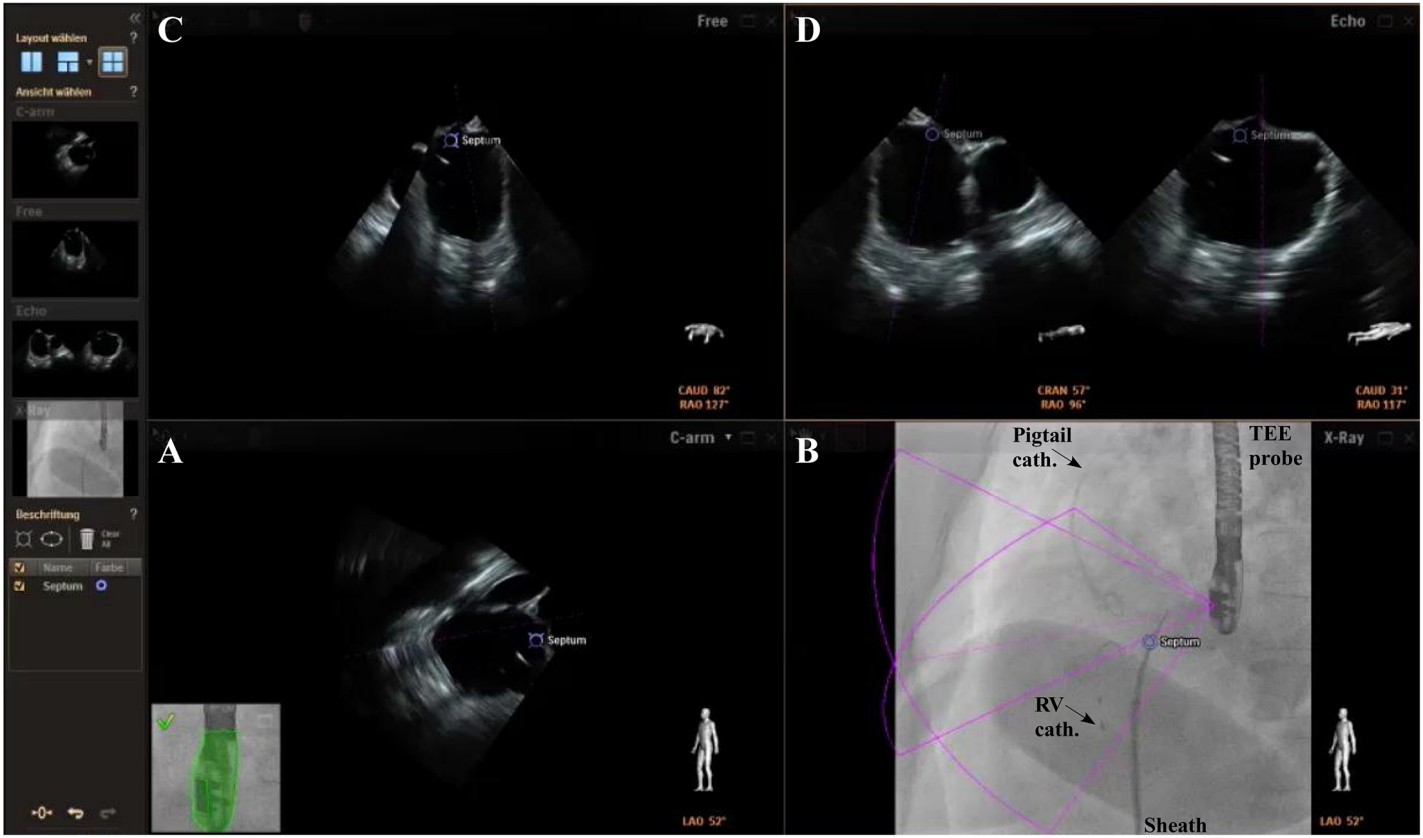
Overview of integrated echocardiography and fluoroscopy imaging. The image acquisition angles during transseptal puncture are depicted in the lower right corner of each image. A+B) Concordant views of TEE and fluoroscopy images. C) In the “Free view” echocardiographic images can be rotated and zoomed independently from the echocardiographer by using a tableside control. D) Conventional echocardiographic view using the x-plane mode for identification of the preferred transseptal puncture site (Septum, blue). Sheath with transseptal needle; Pigtail cath. = pigtail catheter; RV cath. = catheter in the right ventricle.
